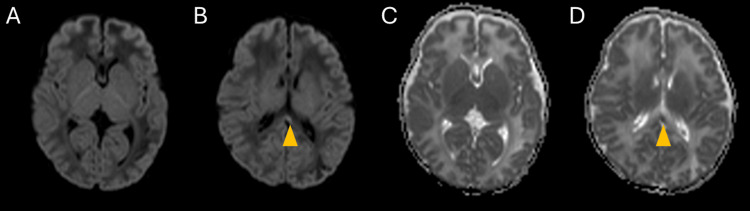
MRI findings on day 14 On DWI, diffusion restriction is mostly resolved (A, B). A part of the lesions that remained in the splenium of the corpus callosum are marked with arrowheads (▲). The mean ADC values of the splenium were 1,298×10^-6^ mm^2^/s (C, D). MRI: magnetic resonance imaging; DWI: diffusion-weighted imaging; ADC: apparent diffusion coefficient
